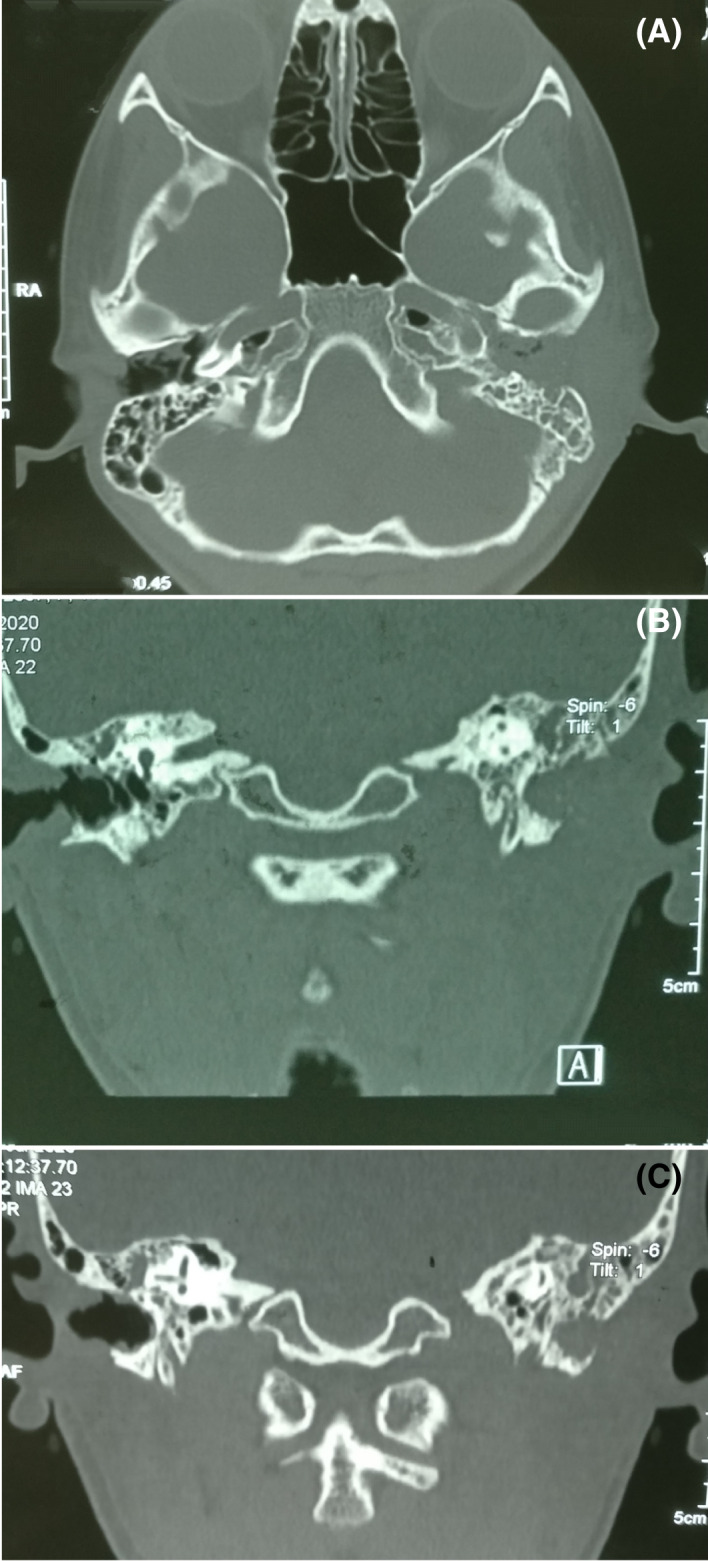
High‐resolution computed tomography (HRCT) scan of temporal bone revealing (A) ballooning of bony external auditory canal with destruction along with soft tissue density in mastoid, (B) soft tissue density in attic with facial canal dehiscence, (C) dural plate dehiscence
